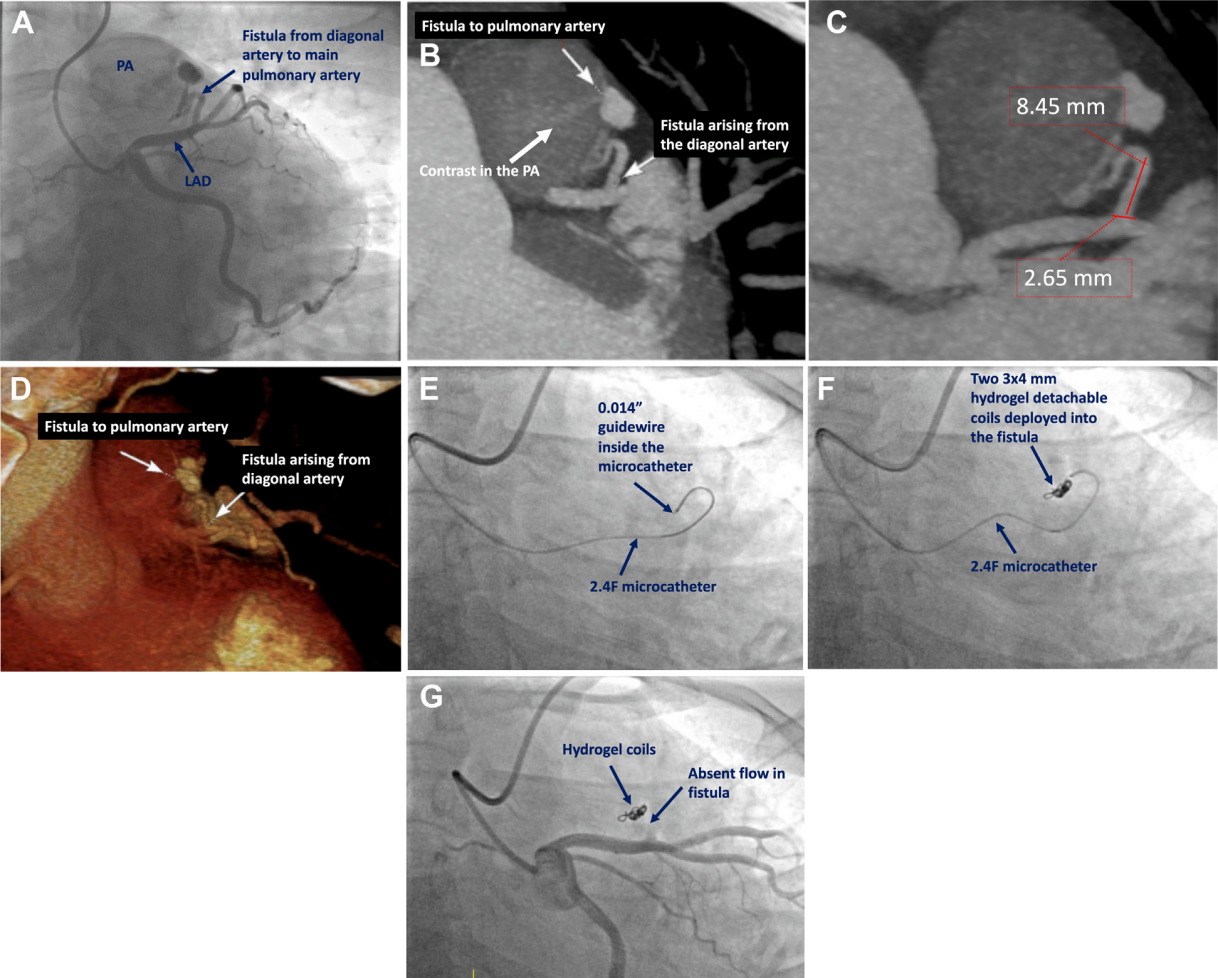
Preprocedural Imaging and Transcatheter Intervention (A) Coronary angiography demonstrates a fistula from the diagonal artery to the pulmonary artery. (B) Coronary computed tomographic angiography demonstrates the fistula from the diagonal artery to the pulmonary artery. (C) Measurements of the length (8.4 mm) and diameter (2.6 mm) of the fistula are used to guide selection of coils for embolization. (D) Three-dimensional volume rendering demonstrates the origin and course of the fistula. (E) The 2.4-F microcatheter is advanced over a 0.014-inch guidewire into the fistula. (F) Two 3 × 4 mm hydrogel detachable coils are deployed into the fistula. (G) Final angiography demonstrates occlusion of the fistula and TIMI flow grade 3 in the left anterior descending coronary artery and diagonal artery.
